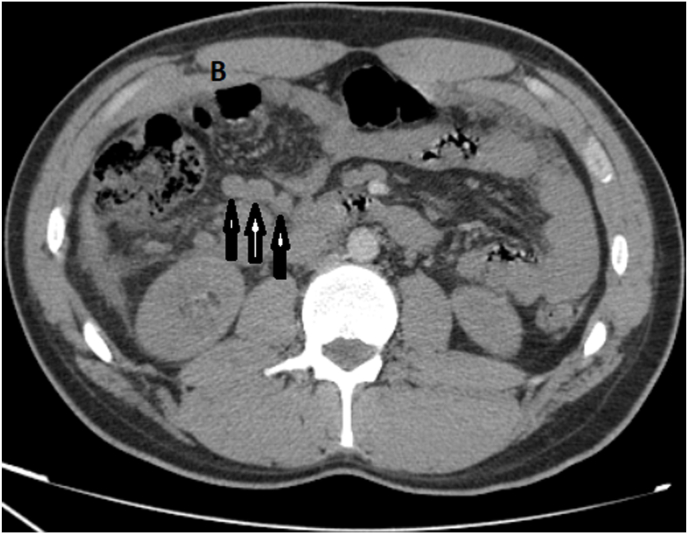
CT scan of abdomen (axial plane) reveal mesenteric and para-aortic lymphadenopathy (three vertical arrows).
